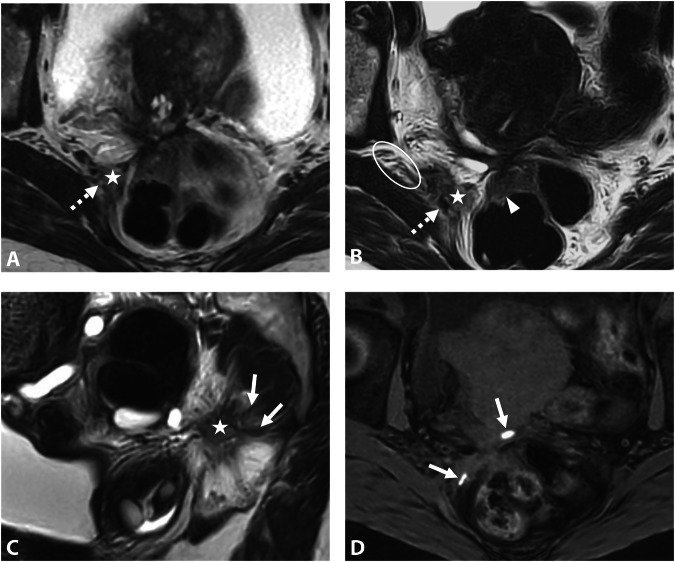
DE in a 42-year-old woman, with a history of rectal shaving 10 years ago, who presented with a recurrence of symptoms, in particular deep dyspareunia and right sciatica. A, B Axial T2 and (C) sagittal T2W MR images show right subperitoneal infiltrative lesion involving the anterolateral rectal wall (B, arrowhead), extending to the right posterolateral parametrium with inferior hypogastric plexus involvement (stars), up to the pelvic wall with encasement of hypogastric vessels (A, B, dashed arrows) and contact with the piriformis muscle. The posterior spiculated margins come into contact with the S3 and S4 sacral roots (C, arrows) and the posterior part of the right sciatic nerve (B, circle). D Axial fat-suppressed T1W MR image reveals T1-hyperintense endometriotic hemorrhagic microcysts (arrows). The surgical procedure confirmed extrinsic involvement and included a shaving of the right sacral roots and right sciatic nerve, as well as a section of the hypogastric vessels
